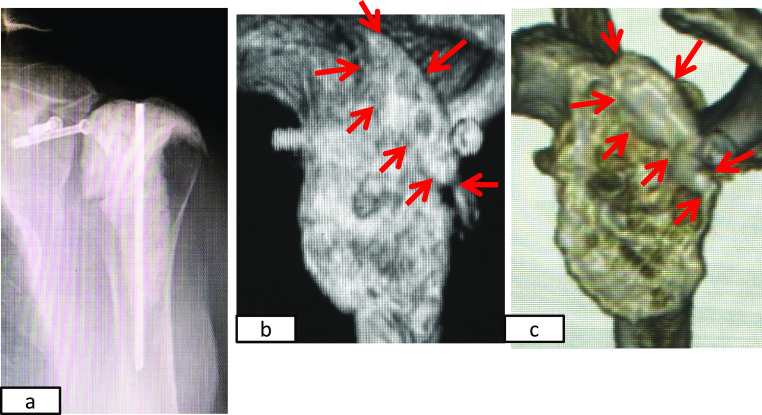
Images obtained after the second operation. a) Radiography showing the two cannulated screws inserted to fix the allogenic
